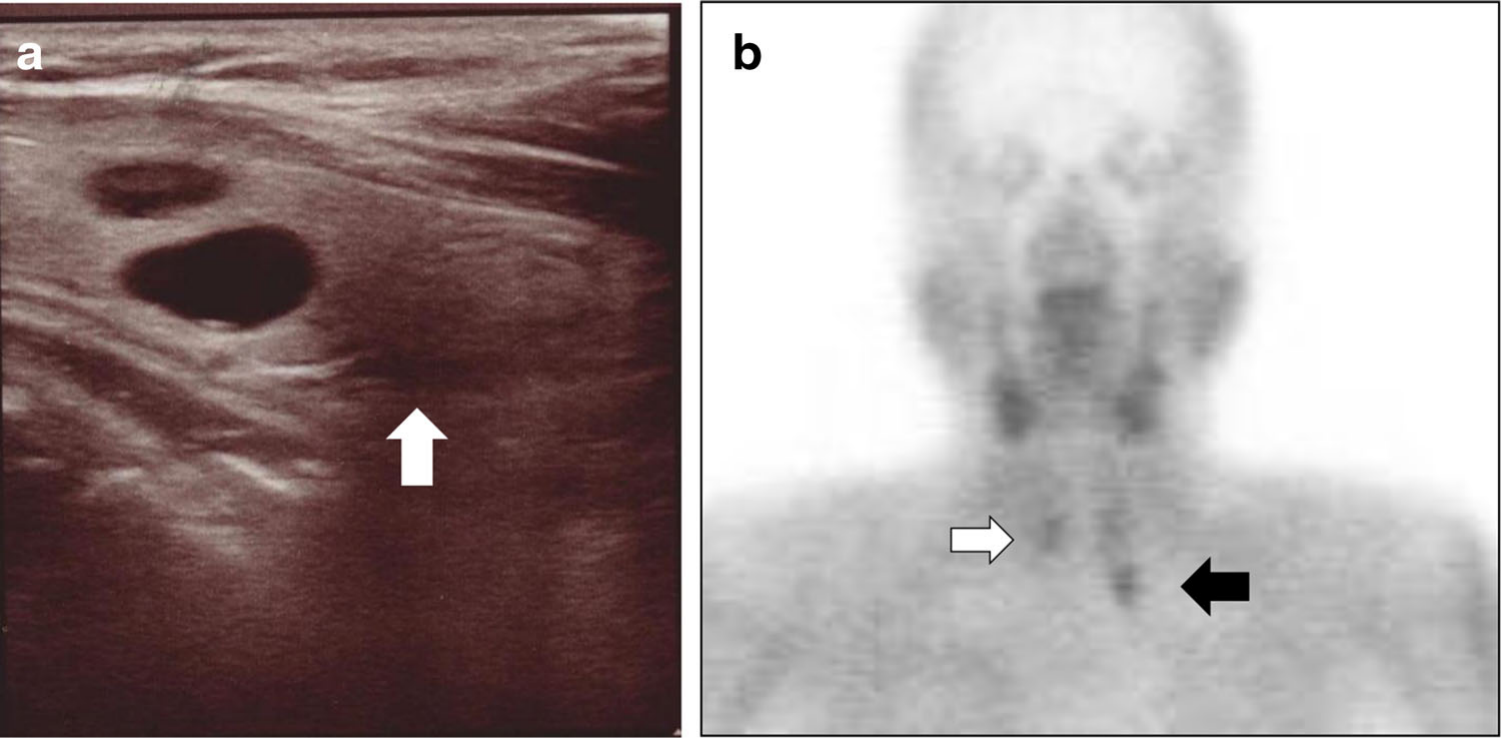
a Neck US exhibited a low echoic mass behind the lower pole of the patient’s left thyroid lobe (*white arrow*). b The delayed phase of the ^99m^Tc-MIBI scintigram indicated abnormal uptake in the left *(arrow*) and right (*open arrow*) neck regions (a)
